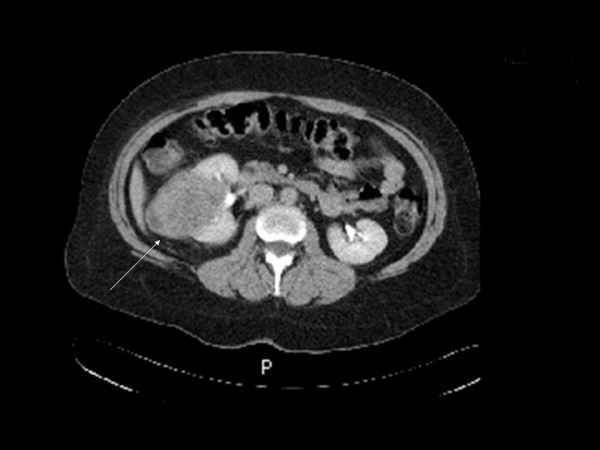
CT image showing the primary lesion in the right kidney.
